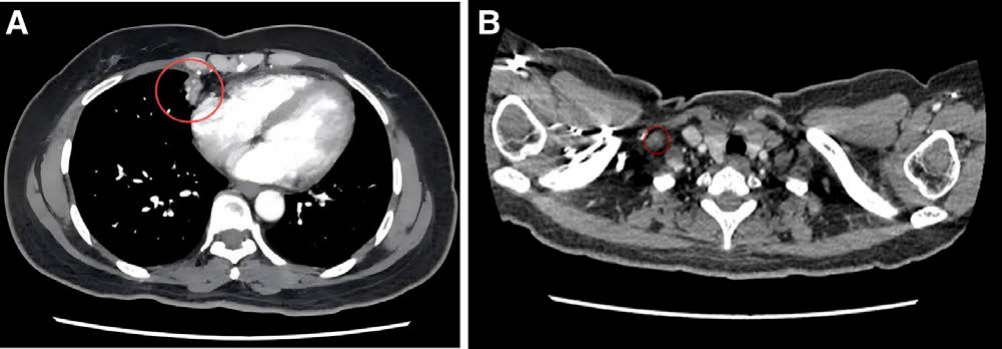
Chest CT follow-up on October 30, 2020. (A) Significant reduction in the right lung lesion compared to the previous scan; (B) significant reduction in the size of the right supraclavicular lymph node. CT = computed tomography.
